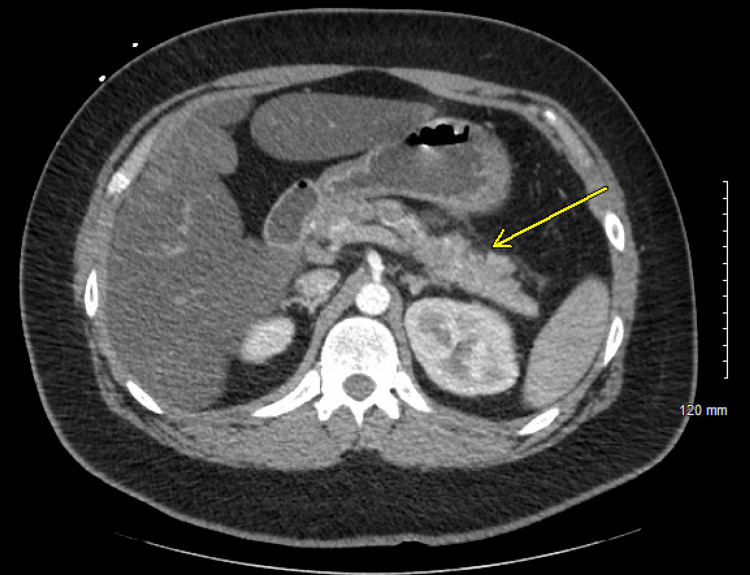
CT abdomen with contrast showing signs of acute pancreatitis
